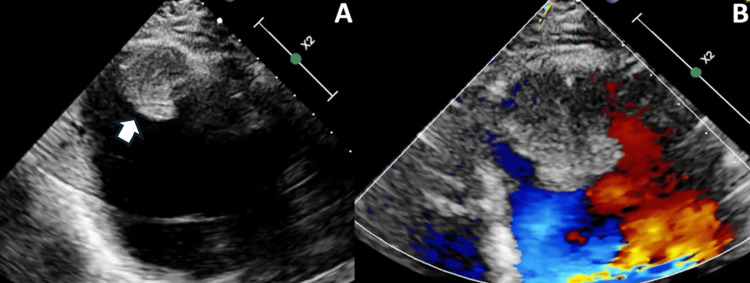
(A) Two-dimensional echocardiography shows an echodense, rounded mass adhered to the left ventricular apex (white arrow). (B) Apical zoom view color Doppler imaging reveals a lack of blood flow within the mass, confirming its solid, thrombotic nature.
